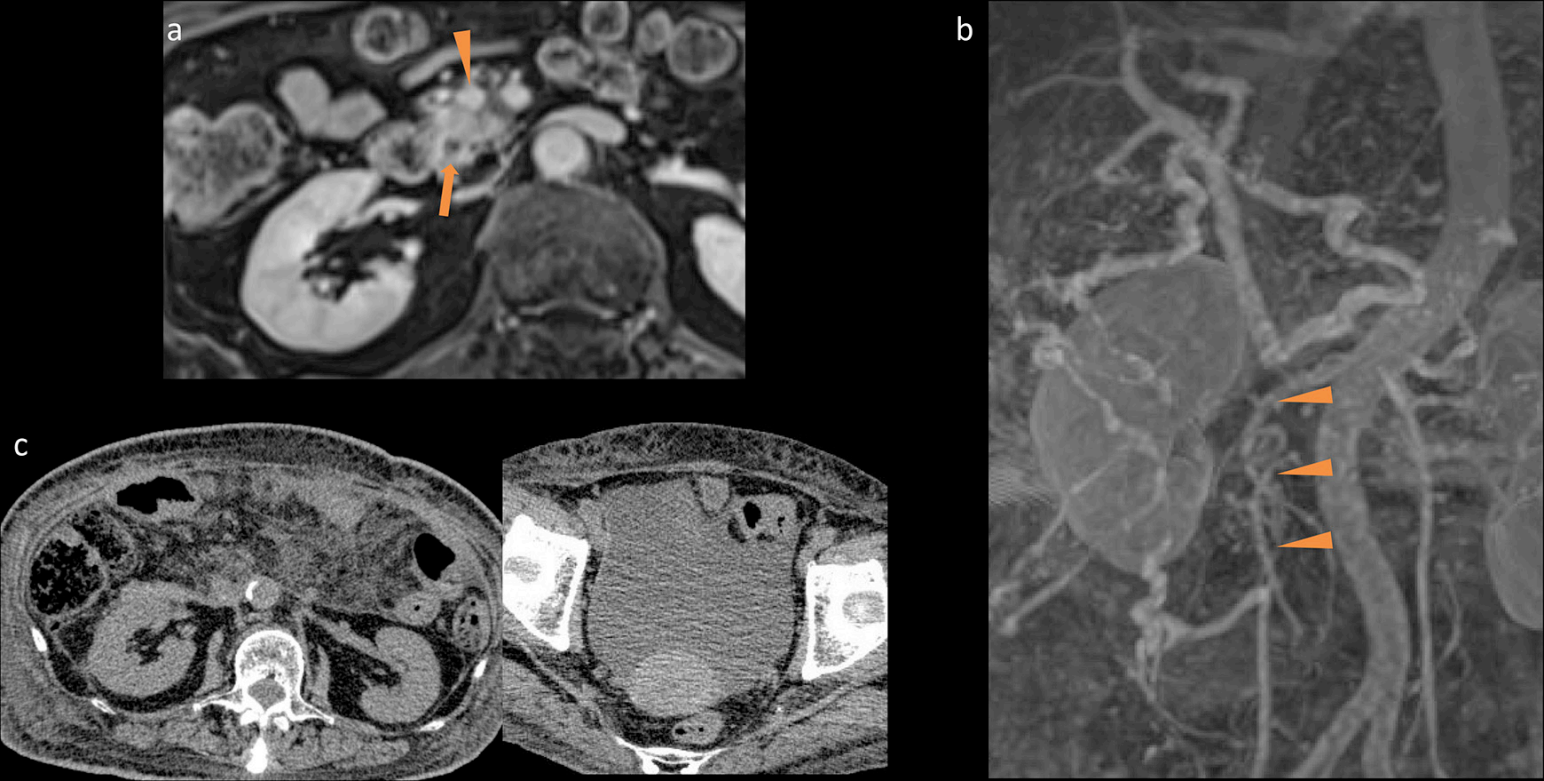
Contrast-enhanced magnetic resonance imaging (MRI). (a) Preoperative contrast-enhanced MRI revealed that the superior mesenteric vein (SMV) (arrowhead) was near the pancreatic cancer (arrow). (b) Postoperative contrast-enhanced MR angiography. A coronal maximum intensity projection image revealed a 6.5 cm portal vein and SMV occlusion (arrowhead). (c) Postoperative computed tomography showed massive ascites and oedema of the lower limbs.
